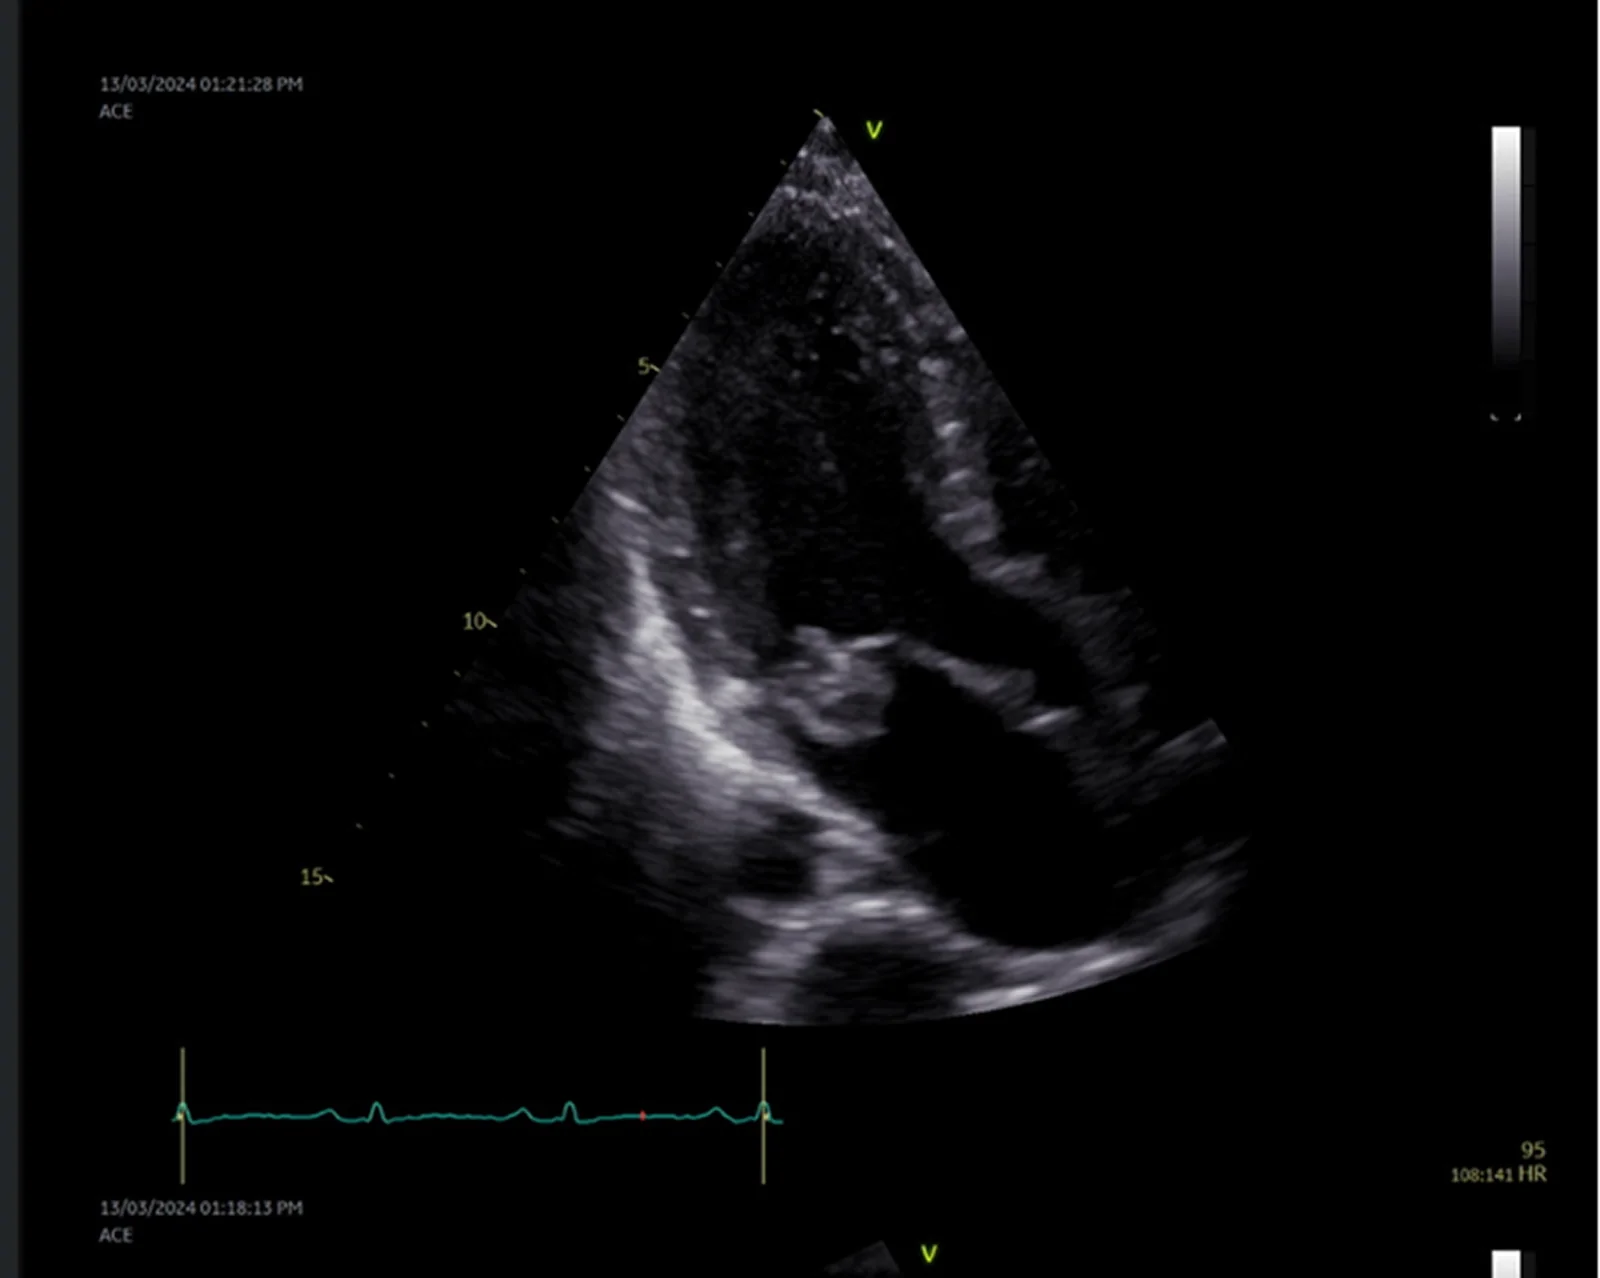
Apical three-chamber view via transthoracic echocardiography demonstrating anterior mitral valve perforation with a small vegetation on the atrial side (Patient 3).
